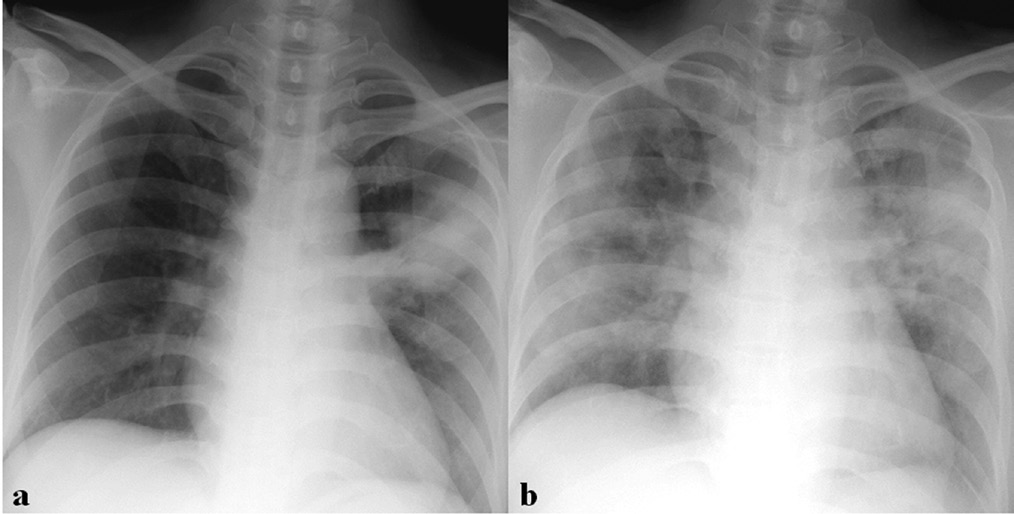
A 36-year-old male patient with SARS-CoV infection. (a) Initial chest radiograph (CXR) shows focal nodular consolidation in left upper lung zone. (b) Follow-up CXR obtained 6 days after (a) shows rapid progression with bilateral diffuse consolidation and GGOs.
